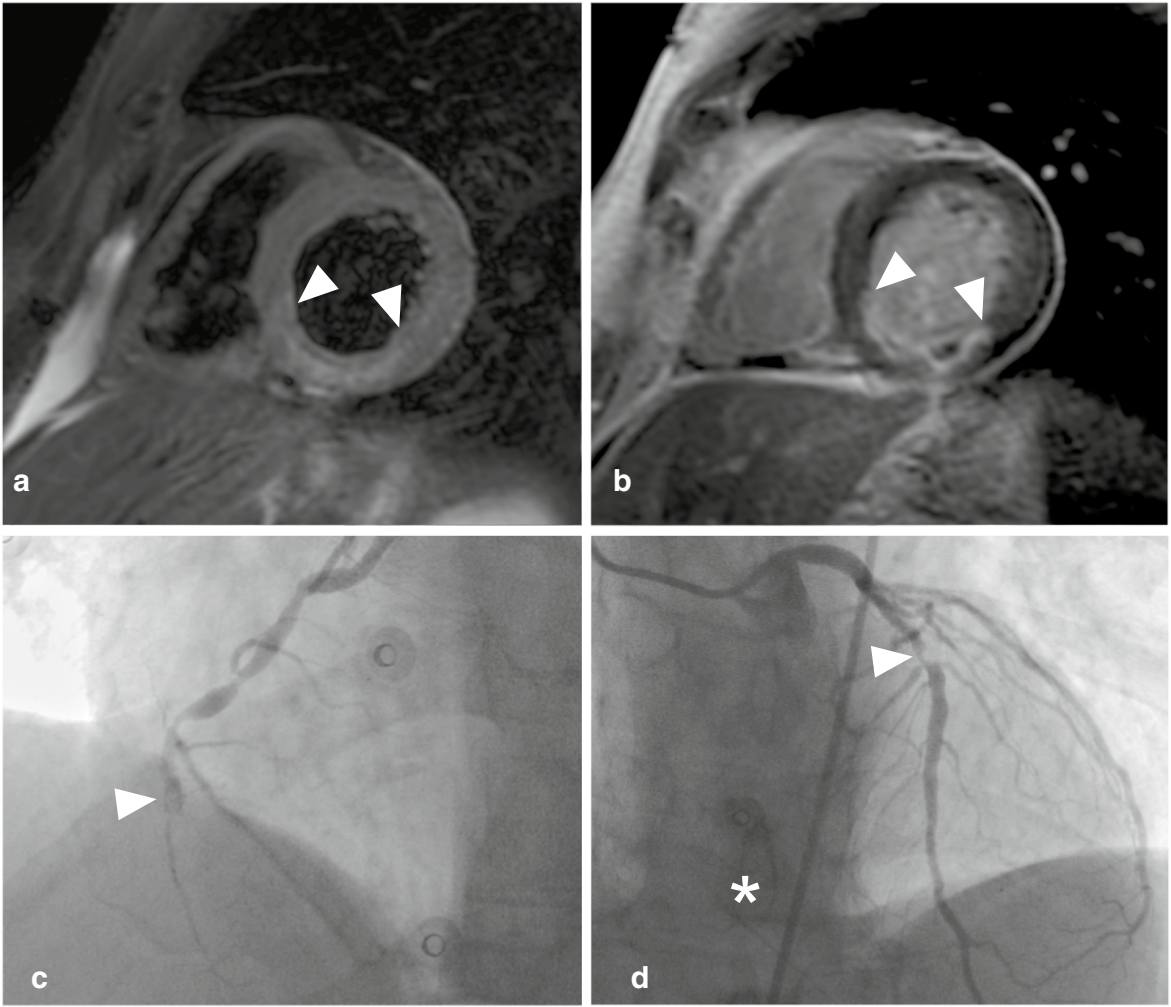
Patient example. Upper row: basal-inferior-inferoseptal edema on T2-weighted short axis left ventricular image (a arrowheads), 50–75% transmural basal-inferior-inferoseptal myocardial infarction with microvascular obstruction (b arrowheads). Lower row: total occlusion of the mid right coronary artery (c arrowhead), 71–90% mid left anterior descending artery stenosis (d arrowhead) with collaterals to right coronary artery (d asterisk)
